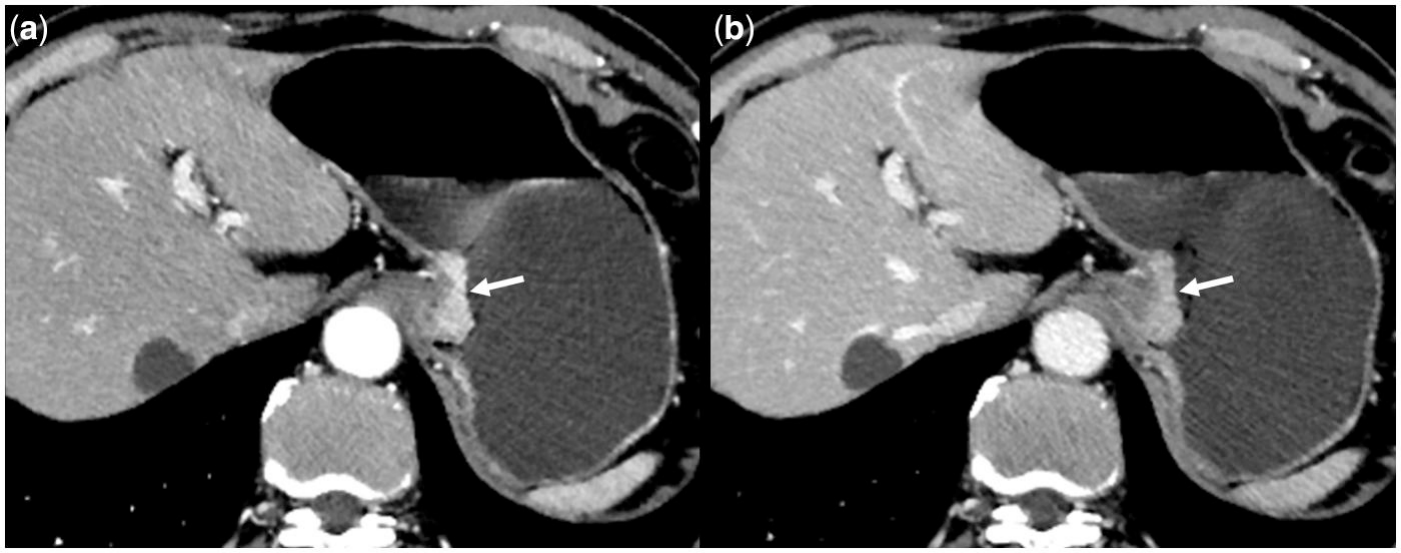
Multi-slice computed tomography scans of a 62-year-old male patient with human epidermal growth factor receptor 2-negative GC. Axial-enhanced arterial phase (A) and portal phase (B) computed tomography images show GC in the lesser curvature of the stomach (arrow), with a well-defined margin, no necrosis, and negative cancer antigen 125. GC = gastric cancer.
